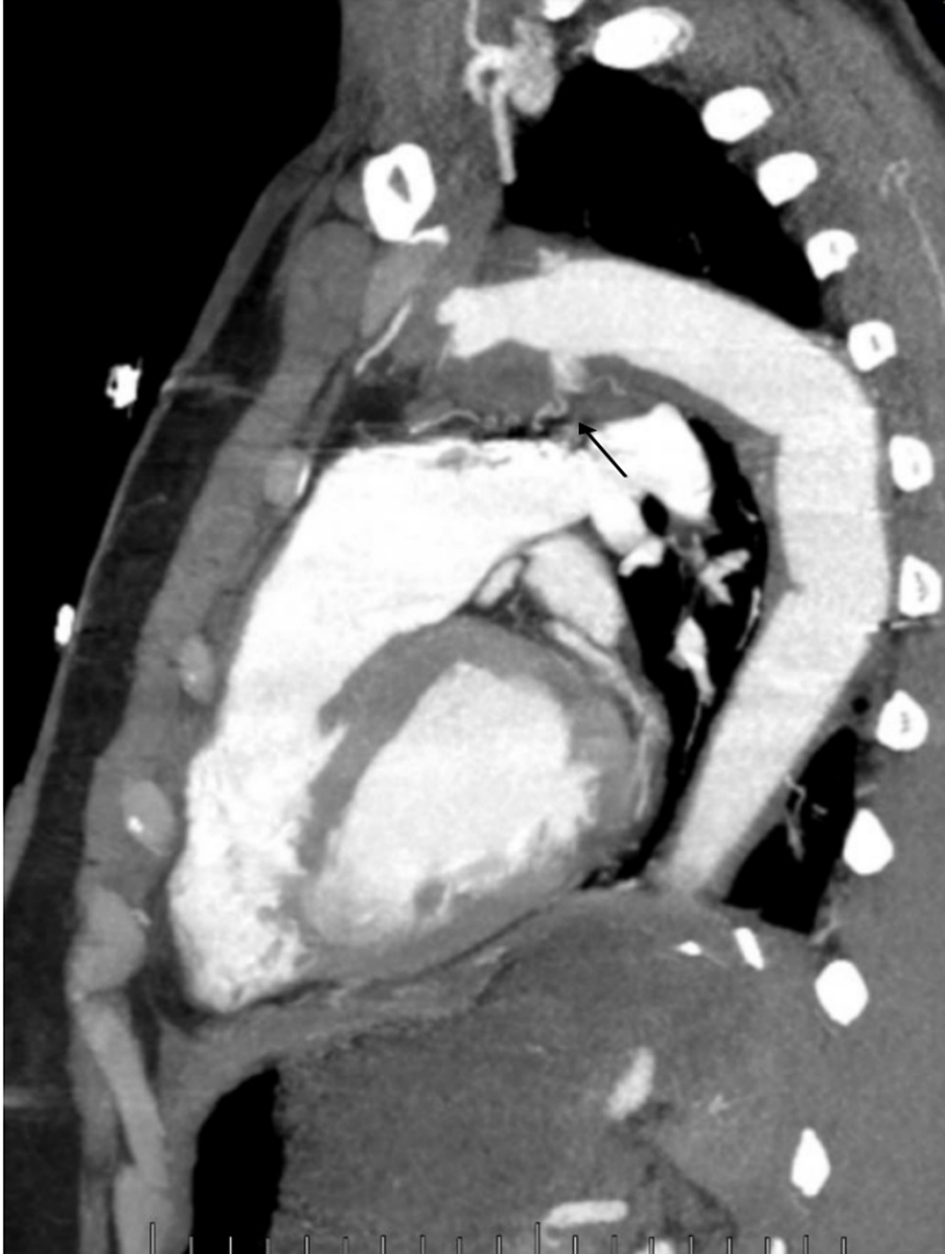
Contrast-enhanced CT of the chest ( sagittal view) The arrow shows the aortic arch with a peri-aortic thrombus.
